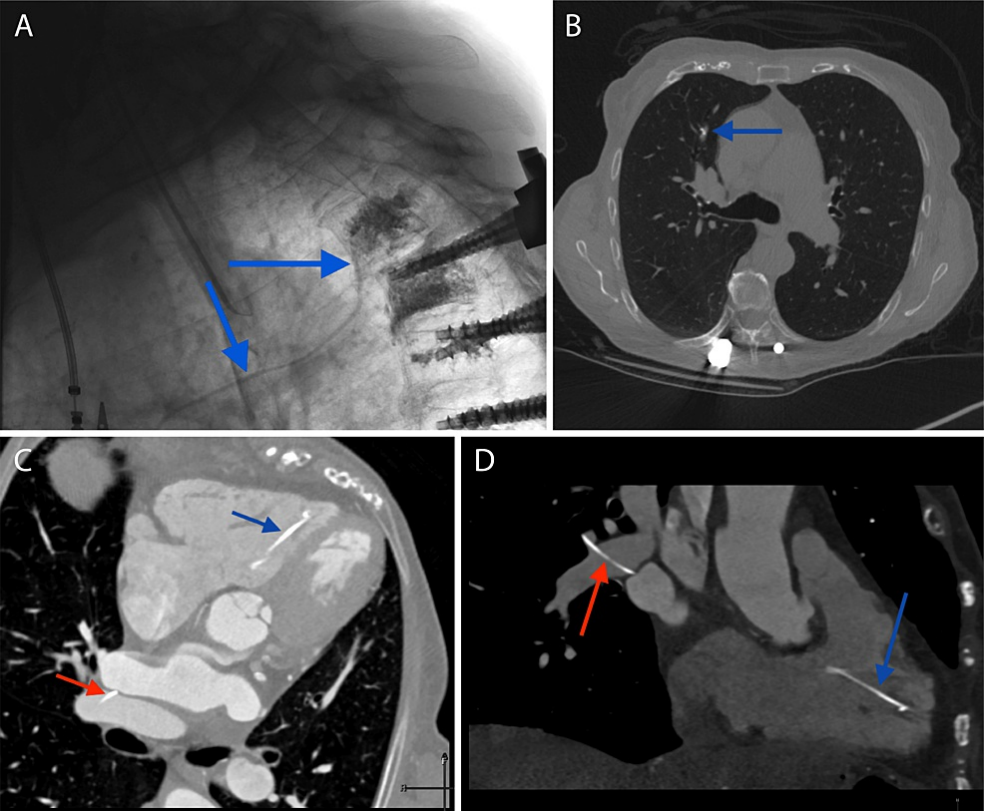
Fluoroscopy and CT imaging depicting cement extravasation and emboli locations (A) The sagittal intra-procedural fluoroscopic image shows radiodense cement emanating from the T3 vertebral body toward the thorax (blue arrows). An endotracheal tube and posterior spinal fusion hardware are partially in the field of view. (B) An axial CT of the chest shows a small focus of cement within a segmental arterial branch of the middle lobe of the right lung (blue arrow). (C) An axial oblique CT image of the heart demonstrates a linear focus of cement within the right ventricle (blue arrow). Some linear cement is seen along the right pulmonary artery as well (red arrow). (D) A coronal oblique CT image of the heart demonstrates a linear focus of cement within the right ventricle (blue arrow). Some linear cement is seen along the right pulmonary artery as well (red arrow).
